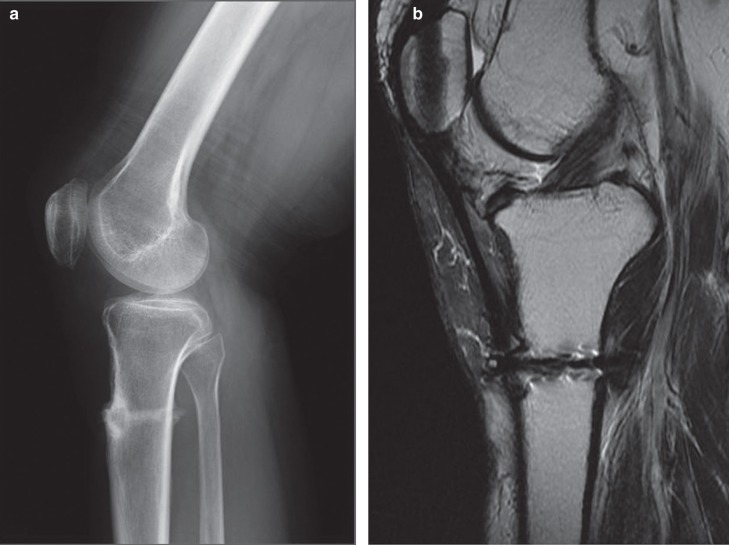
Lateral-view radiograph (a) and MRI (b) taken 3 years after the operation. Bone union is obtained well and the Insall–Salvati index is 1.6 (the opposite knee is 1.1). Therefore, the patella alta is seen. The graft is shown as a dark band on the T2-weighted image (b).
